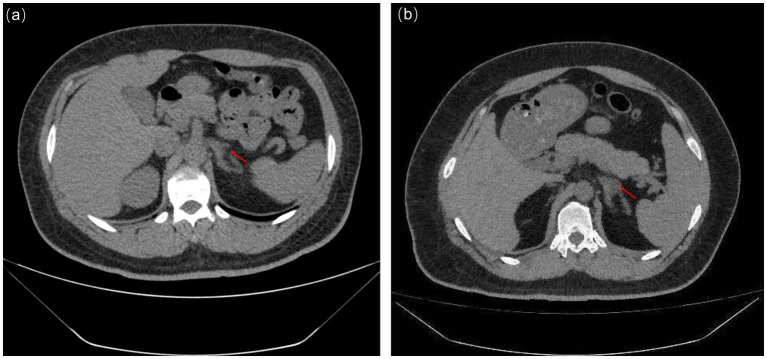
CT scan of left adrenal gland in June, 2019 (a) and September, 2023 (b).
